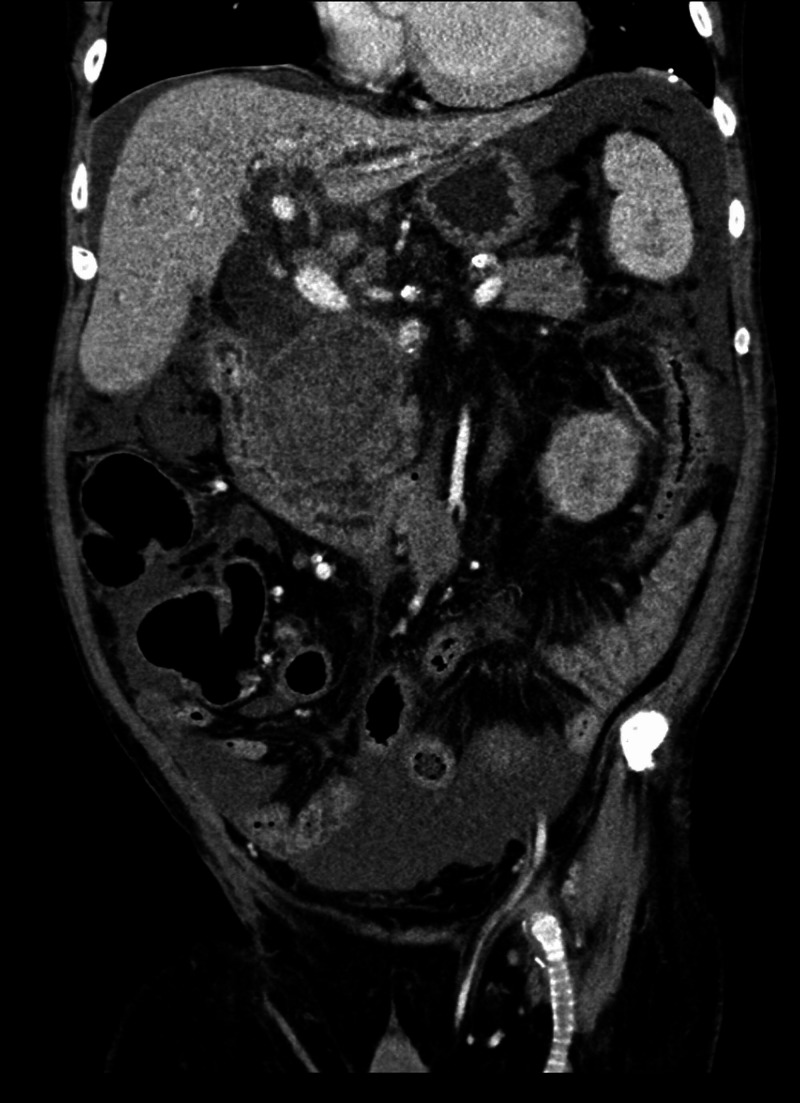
CT with angiogram of abdomen and pelvis (coronal view). The coronal view of the same CT angiogram as Figure 2. The large hematoma is again visualized. Within the liver, intrahepatic biliary dilatation can be appreciated.
